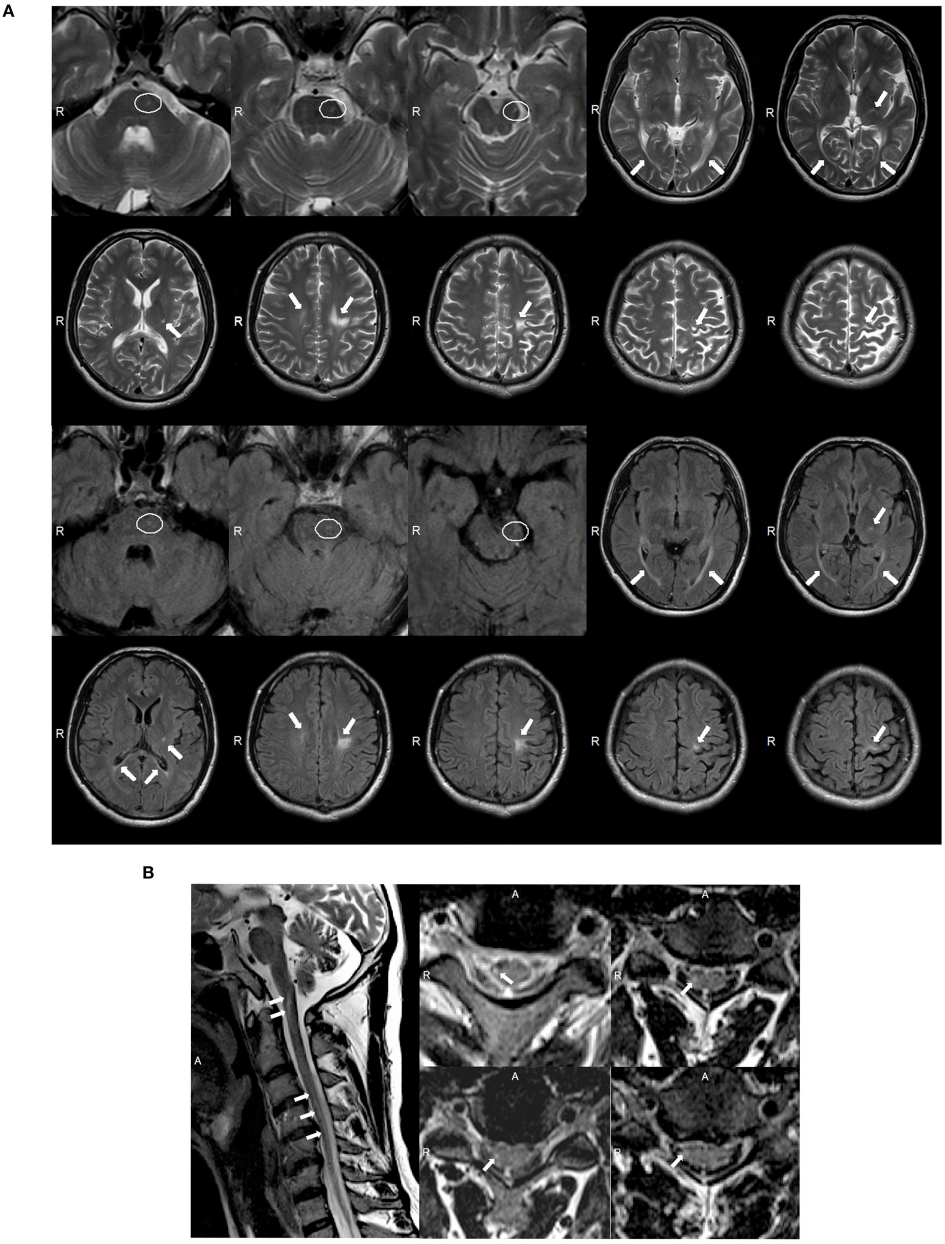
(A) Magnetic resonance imaging (MRI) of the brain for case 2 showed abnormal asymmetric consecutive hyperintensity along the bilateral corticospinal tracts (from the precentral gyrus down to the pons on the left and in the level of the centrum semiovale on the right), and in the optic radiation and corpus callosum on T2-weighted (T2W) and T2W fluid-attenuated inversion recovery (FLAIR) images (white arrowheads). MRI images of the mesocephalon have been enlarged to highlight the lesions on the pons (white ovals). (B) MRI of the spinal cord of case 2 showed abnormal inconsecutive intramedullary lesions from C1 to C5 on T2W images (white arrowheads).
